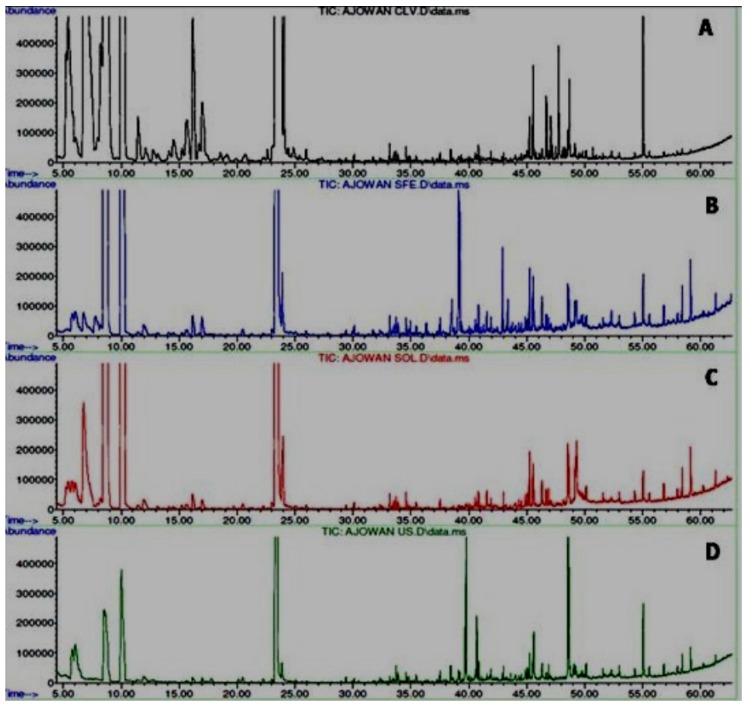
Comparative GC-MS chromatograms of different oils of *T. ammi* L. extracted using different extraction techniques. (A) Hydrodistilled oil, (B) Solvent extracted oil, (C) Ultrasonicated oil, (D) SC-CO_2_ oil.
